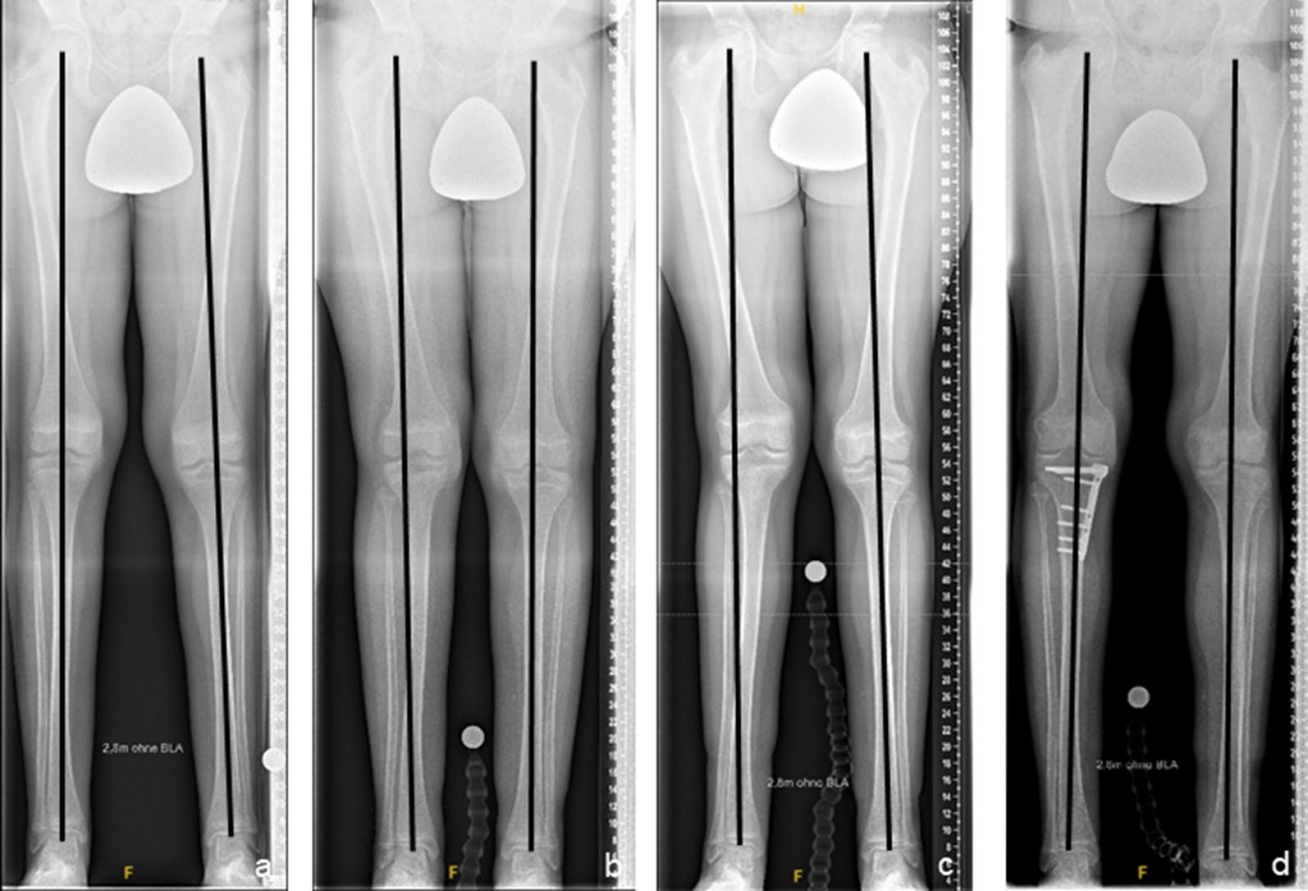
Secondary coronal malalignment after bilateral permanent epiphysiodesis using a bone trephine (EpiStop™) around the knee in a boy with secondary tall stature due to Marfan syndrome. Anteroposterior long standing radiographs right before surgery at the age of 10 years (a), 1 year (b) and 2 years (c) after surgery, showing progressive iatrogenic tibial valgus deformity on the right side, and 2 years after closing wedge correction osteotomy using a locking plate close at the age of 14 years (d), showing physiological coronal realignment and equal leg lengths
